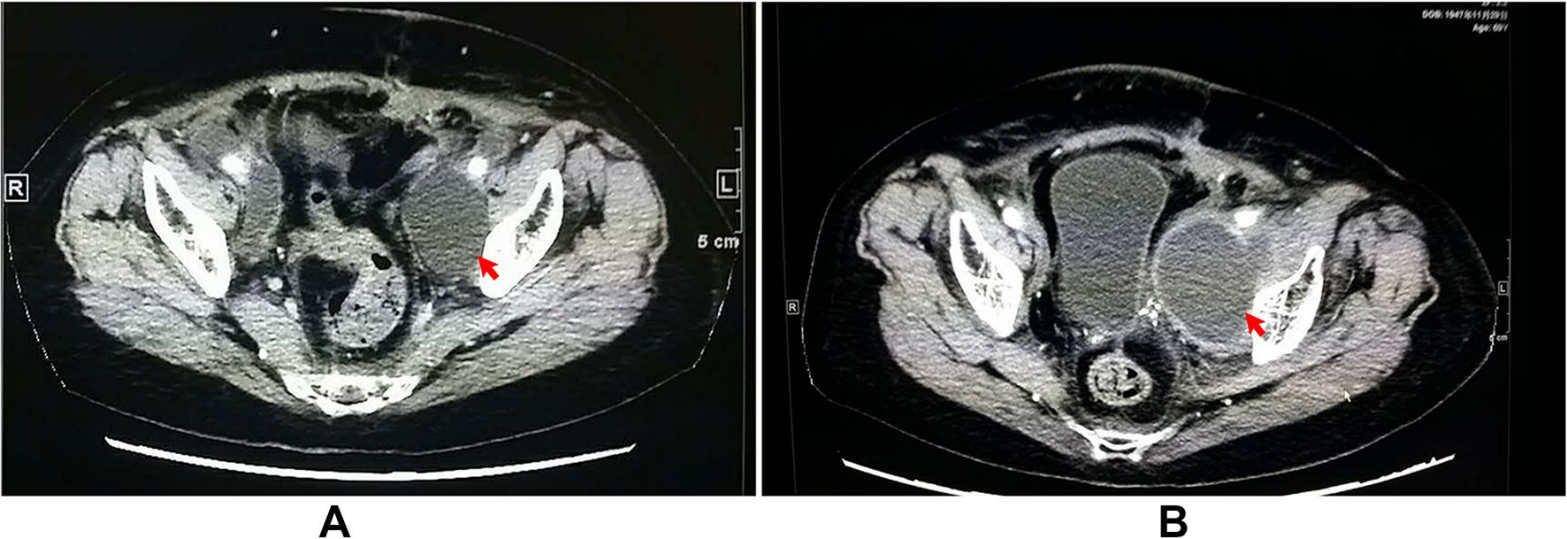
A lymphocele scanned by CT revealing a smooth and thin-walled cavity filled in water-equivalent fluid (a); the same lymphocele became infected and showed low-attenuation with thickened enhanced wall in the same position (b)
